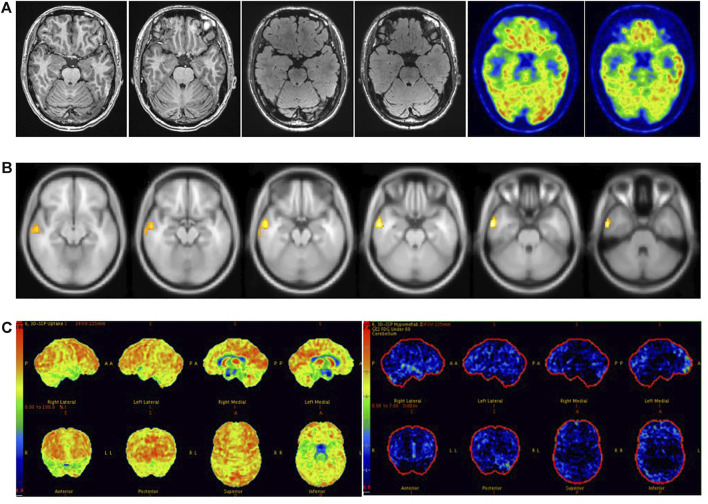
Male, 37 years old, right temporal lobe epilepsy. (A) No structural lesions causing seizures were found at 3.0 T epilepsy-protocol. ^18^F-FDG PET obtained abnormal decreased metabolism in the right temporal lobe. (B) SPM showed decreased metabolism in right temporal lobe while *p* < 0.005 and K > 200. (C) Abnormal metabolism in the right temporal lobe was found by 3D-SSP. The right anterior temporal lobe was surgically resected and remained seizure-free for 2 years follow-up.
